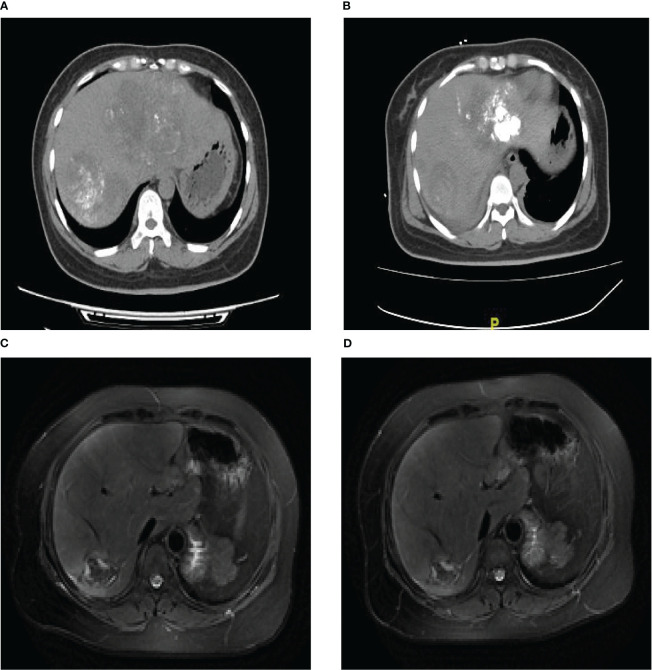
TACE perioperative imaging results. (A) CT scan picture of patient after the first TACE. (B) CT scan picture of patient after four TACE surgeries. (C) The abdomen conventional dynamic enhanced MRI image in March 2022. (D) The abdomen conventional dynamic enhanced MRI image in September 2022.
